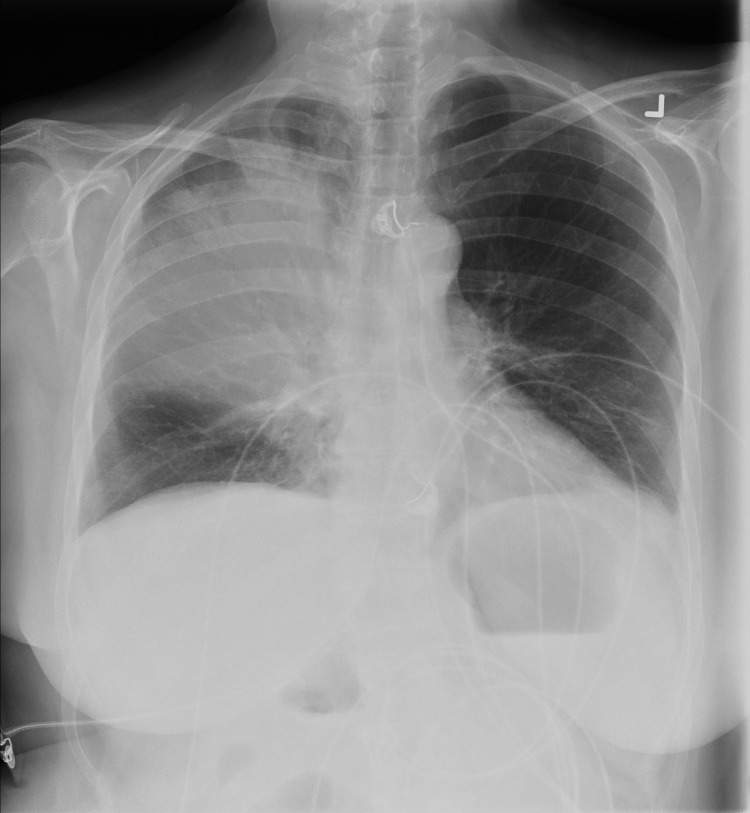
Chest radiograph demonstrating right-upper lobe consolidation in a 67-year-old woman with *Legionella pneumophila* serotype 1 pneumonia.
